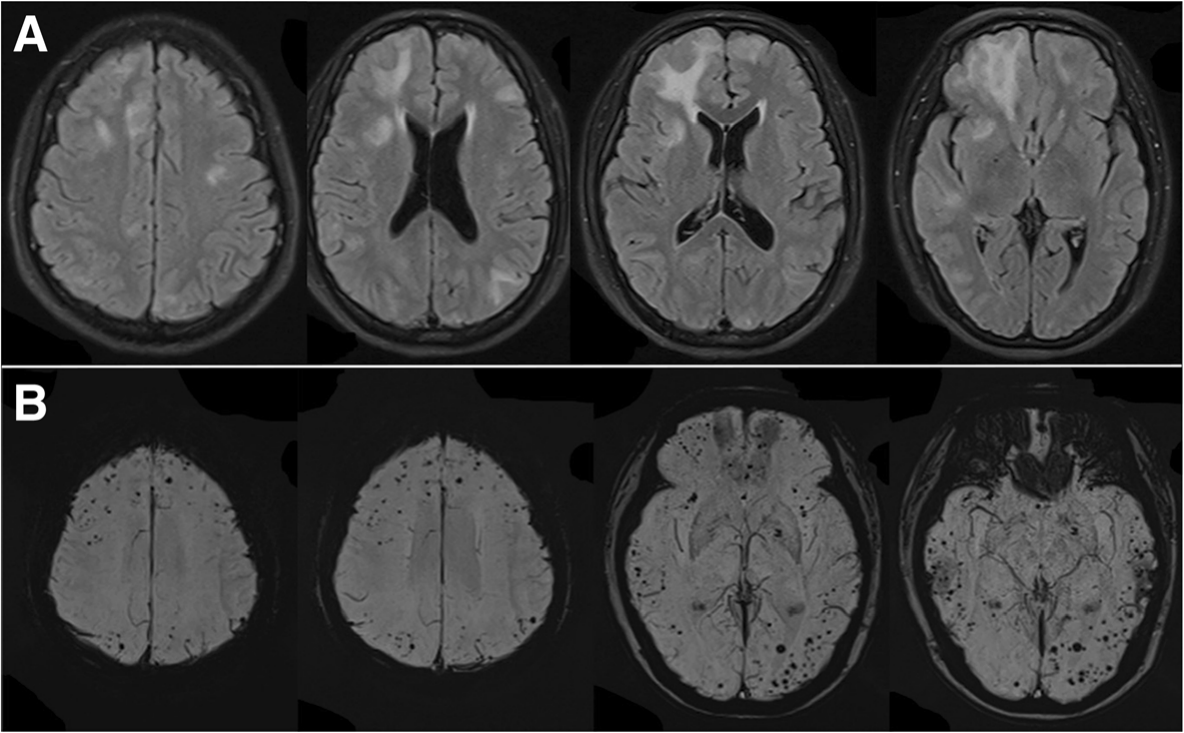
Recurrence MRI images after corticosteroid withdrawal for three months. a multifocal white matter hyperintensity lesions were revealed on FLAIR; b multiple cerebral microbleeds in cortical and subcortical areas increased on SWI
